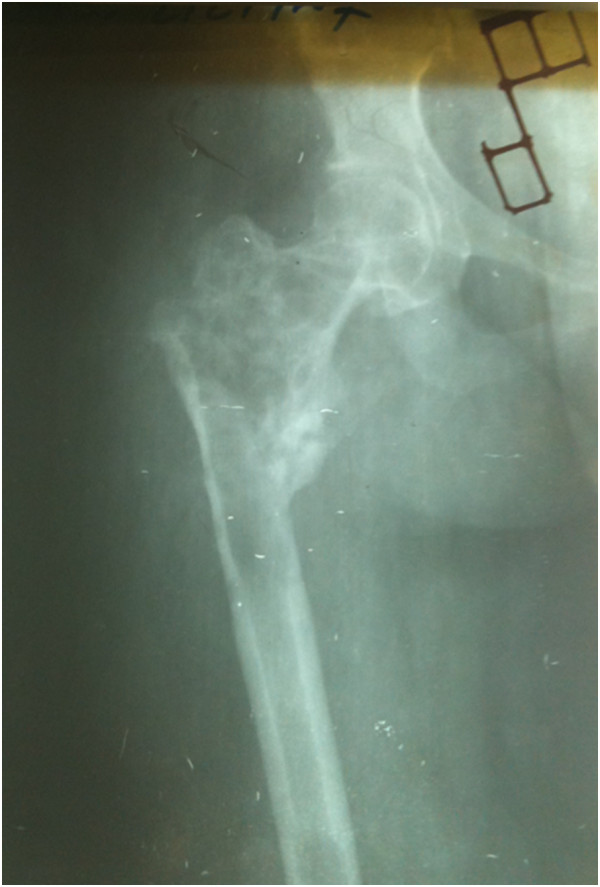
Initial X-ray of the patient before surgical intervention in 1998. There is proximal femur fracture along with radiolucencies present in proximal femur including the femoral head.
